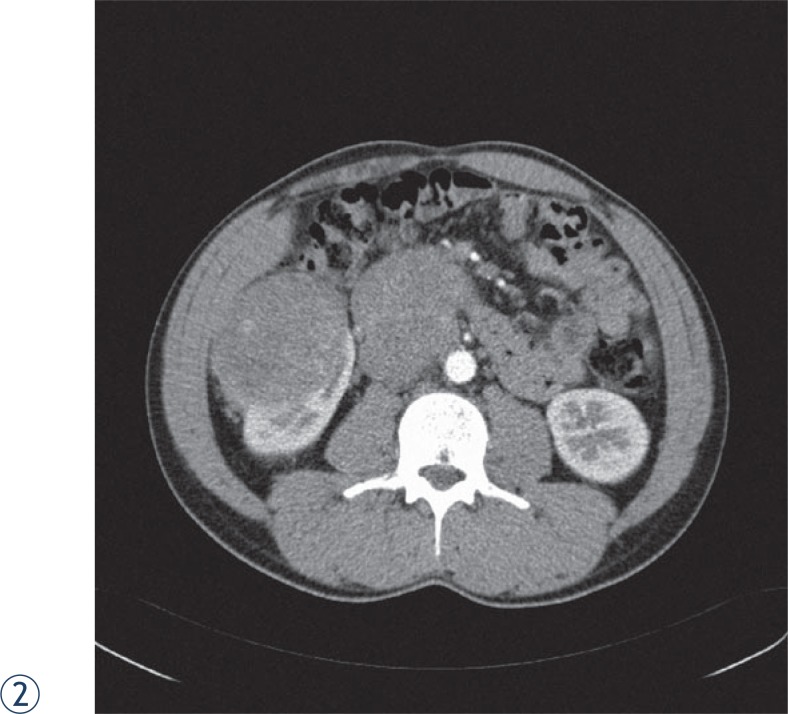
Abdominal CT scan of 27 year adult male.
